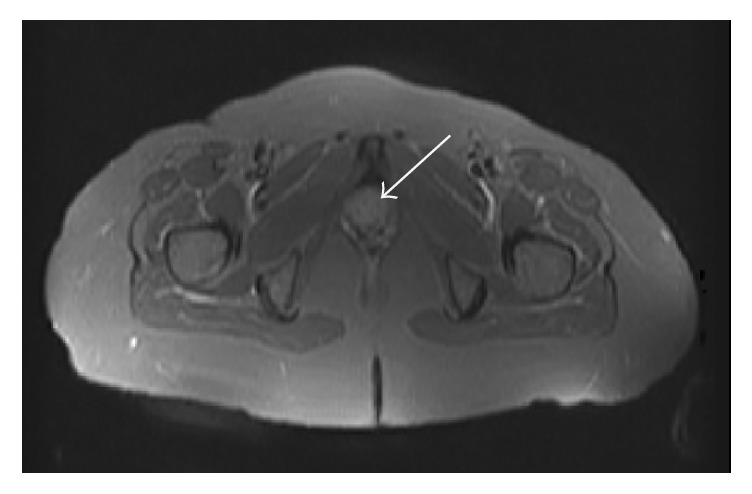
Axial plane pelvic MRI showed an irregular mass with crenulated margins occupying the urethra and vagina.
